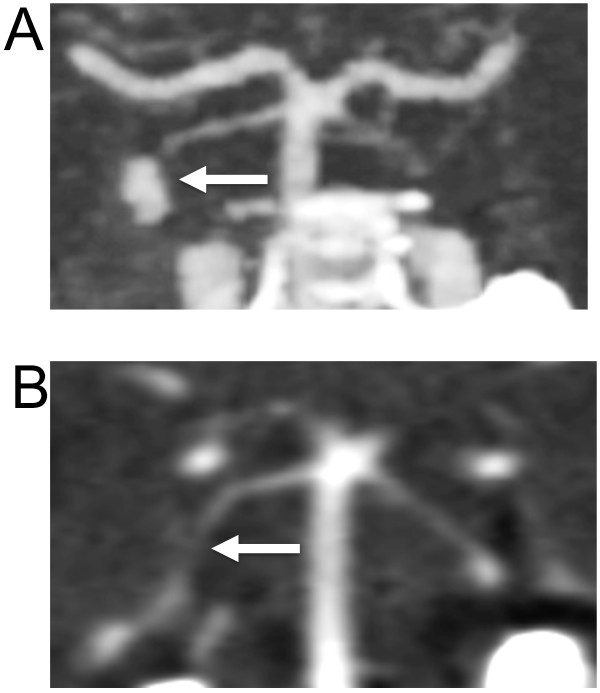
Resolution of superior cerebellar artery aneurysm. CT angiography from neck coronal slices in October, 2012 A. showing prominent aneurysm (arrow) involving the right superior cerebellar artery with B. follow-up on August 2013 indicating resolution of the aneurysm (arrow).
